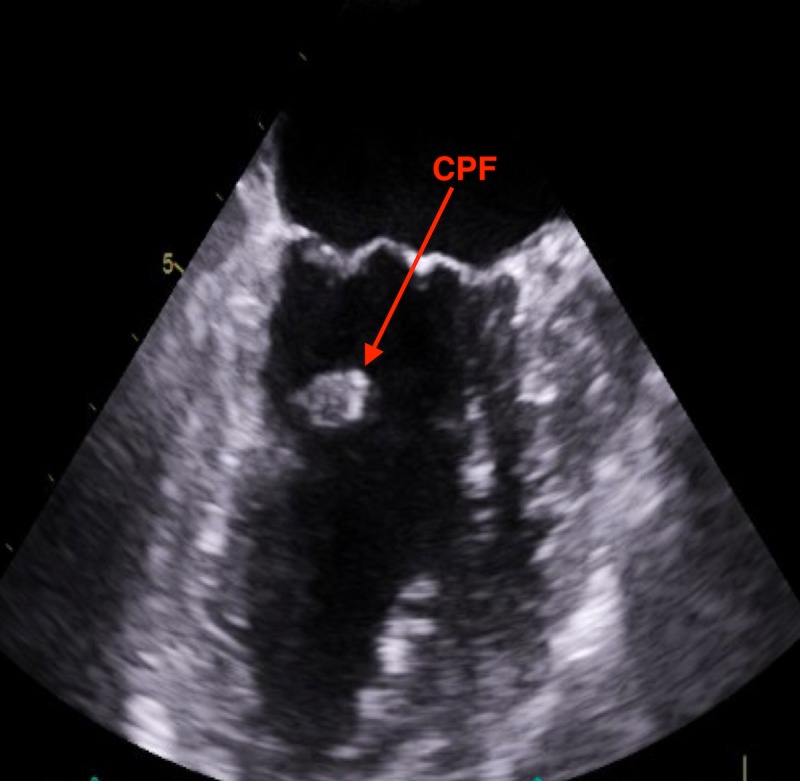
TEE showing mass in the subchordal apparatus of the anterior mitral leaflet TEE: transthoracic echocardiogram
